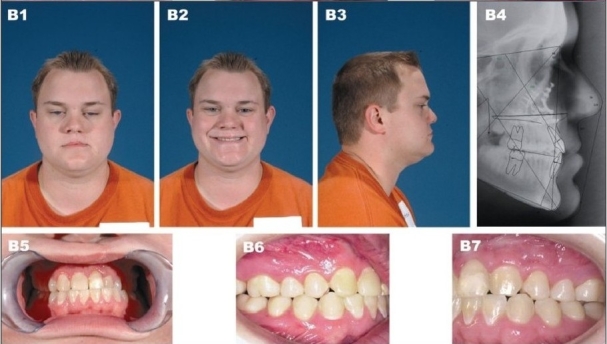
(B) Patient at 23 years of age demonstrating long-term outcome after Lefort I osteotomy with maxillary advancement. (B1) Frontal view (B2) Frontal smiling (B3) Right lateral view (B4) Postperative lateral cephalometric roentgenogram (B5, B6, B7) Occlusion after completion of treatment
